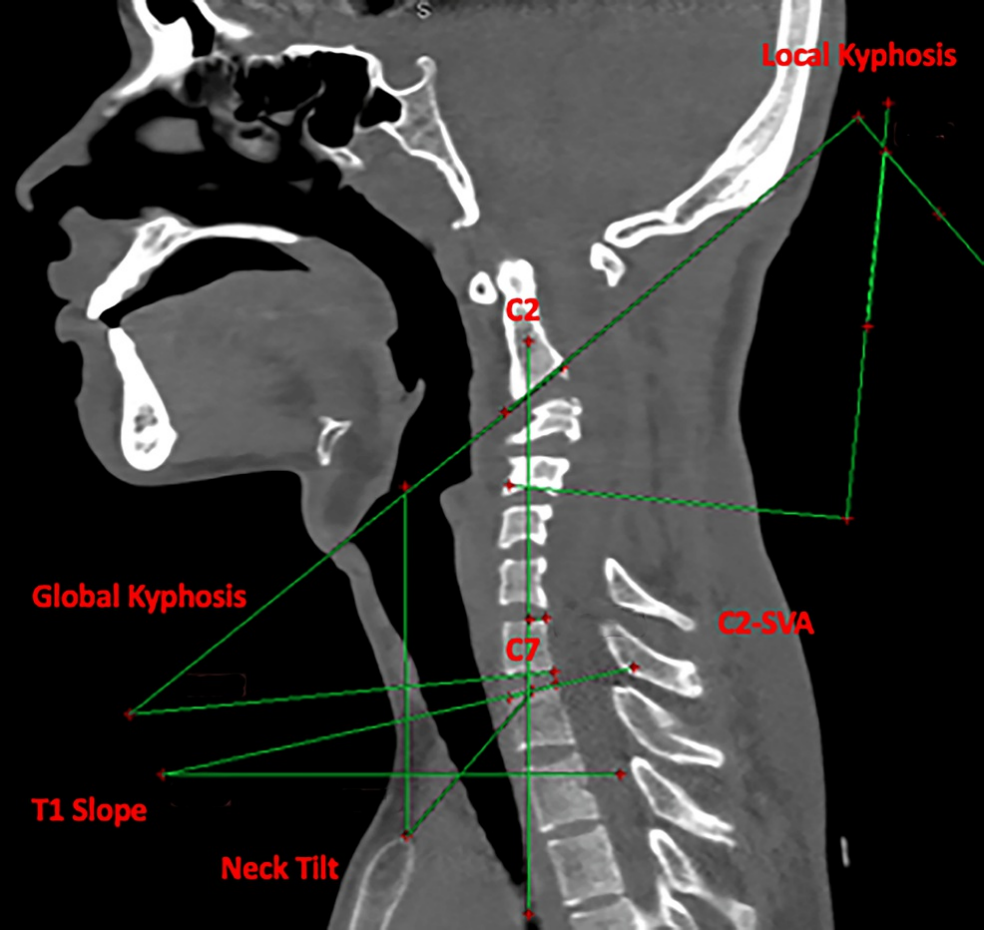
CT sagittal view showing pre-operative assessment of various cervical parameters: local kyphosis 47.4 degrees, global kyphosis 32.9 degrees, neck tilt 41.2 degrees, T1 slope 12.7 degrees, and C2-SVA 4.35 mm. C2-SVA, C2 sagittal vertical axis
